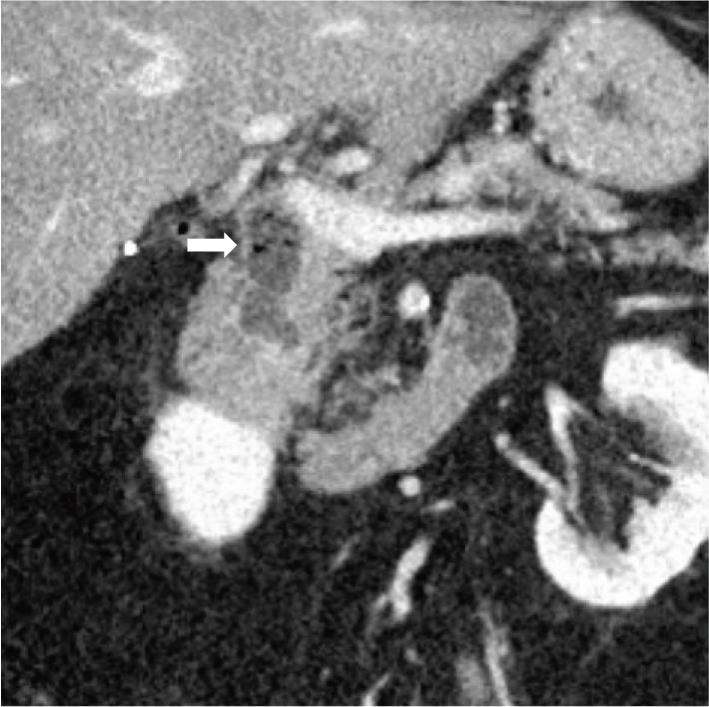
CT scan of the abdomen with arrow indicating dilated intrapancreatic CBD with accumulation of debris and stones, mimicking a duodenal diverticulum.
